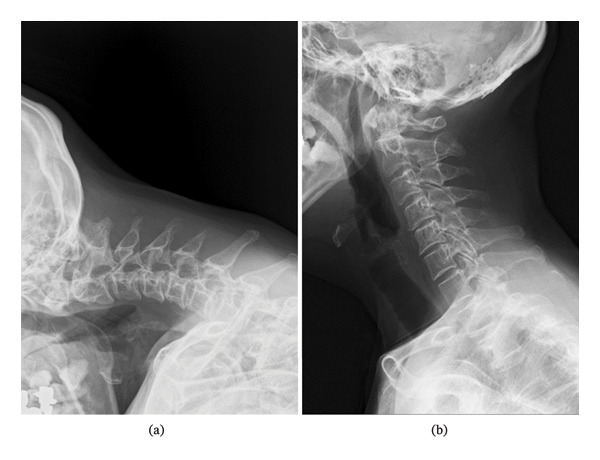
Lateral cervical radiographs. Before the surgery for the aneurysm, chin‐to‐chest phenomenon is observed, indicating dropped head syndrome (a). Dramatically improved cervical extension right after the surgery (b).
